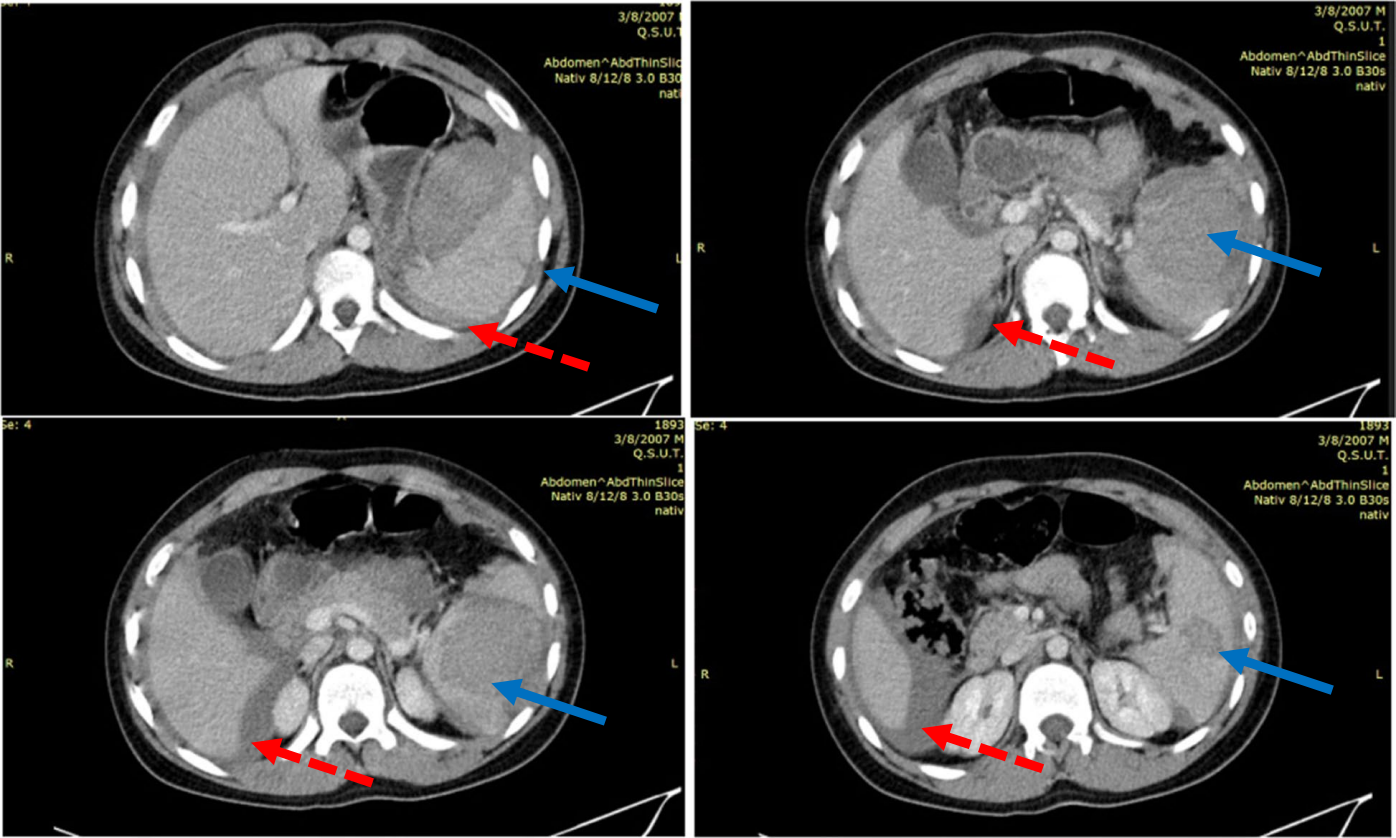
Abdominal CT: There is an intraparenchymal hematoma/laceration measuring > 6 cm which extends to the splenic hilum. (blue solid line) Multiple splenic lacerations were noted inferiorly. No active bleeding on delayed phases. An associated large amount of high density free intraperitoneal fluid/hemoperitoneum around the spleen and liver. (red square dot line) No bone fractures or injuries to other organs
